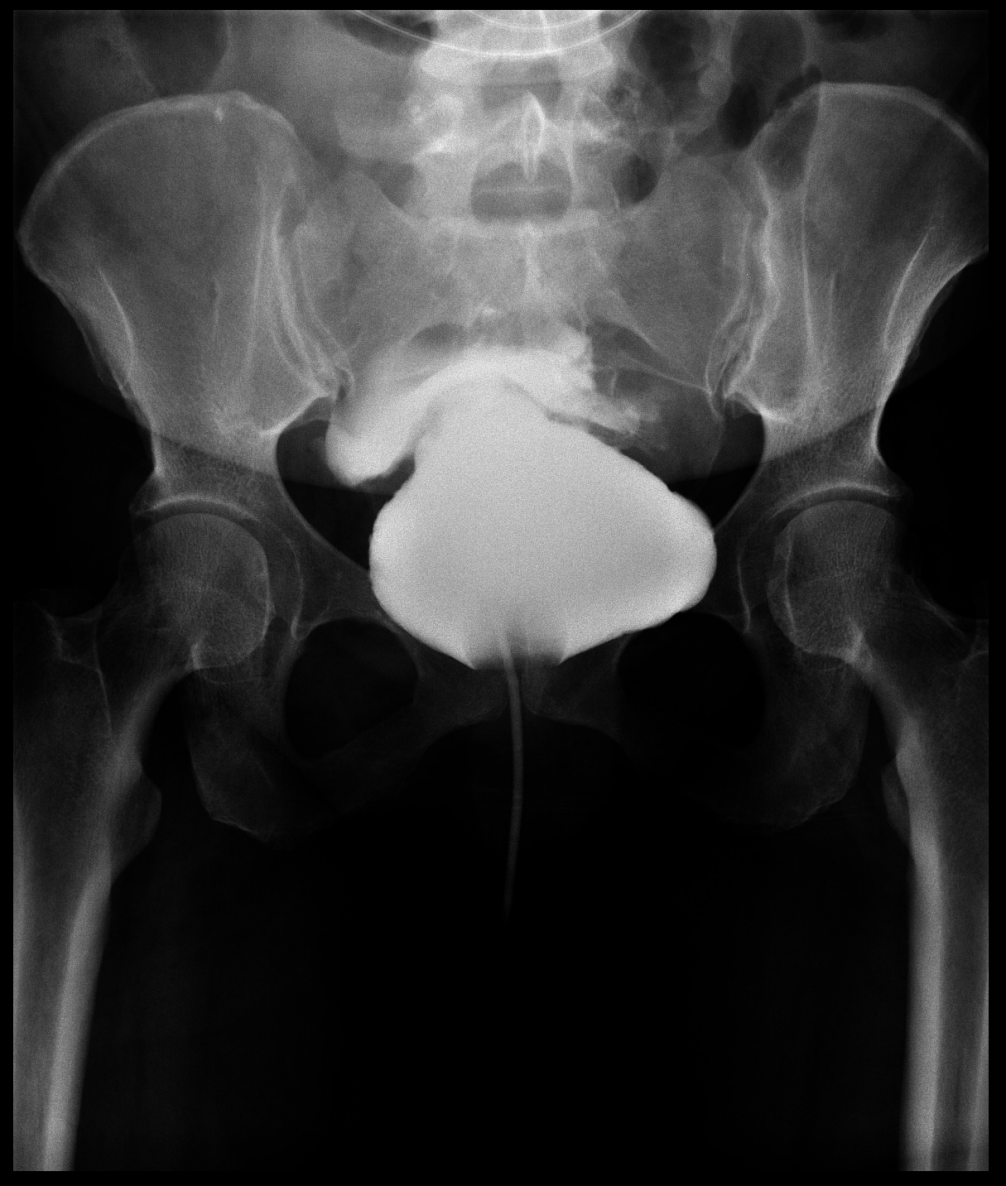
X-ray cystogram showing bladder rupture
